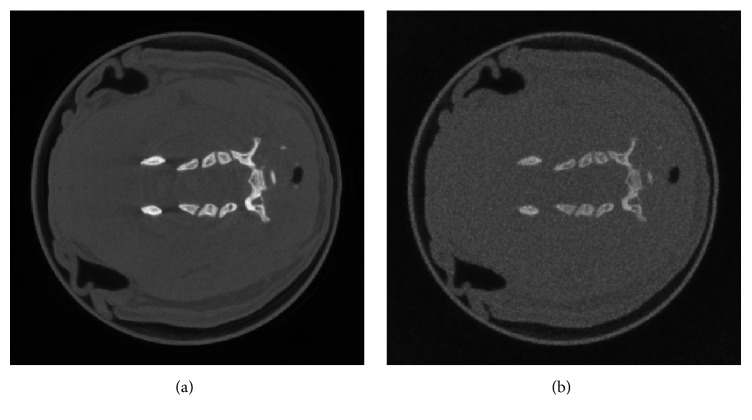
Reconstructed CT images at one slice. (a) Image at energy of 15-60keV, (b) Image at energy of 60-100keV. Display window: [0 0.5].
